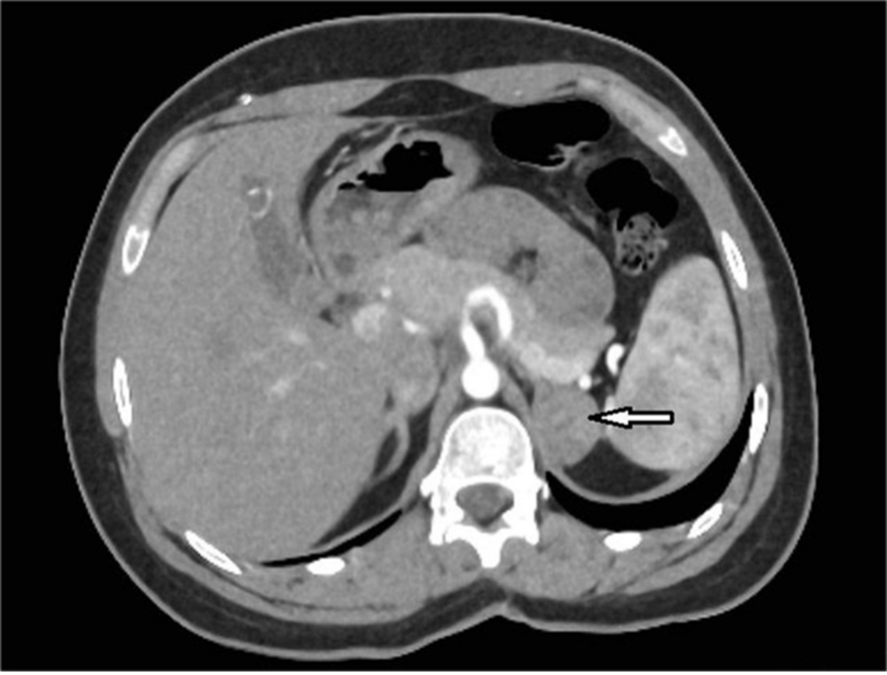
An axial abdominal computed tomography (CT) scan with intravenous contrast administration of case 1 revealed an indeterminate mass communicating with the left adrenal gland measuring approximately 28 x 21 mm (arrow).
